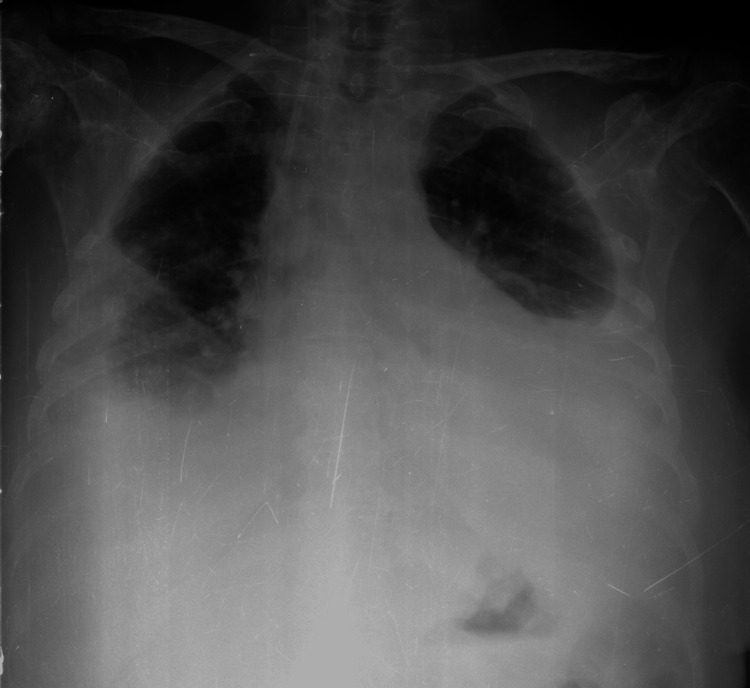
Chest x-ray showing a bilateral pleural effusion.
